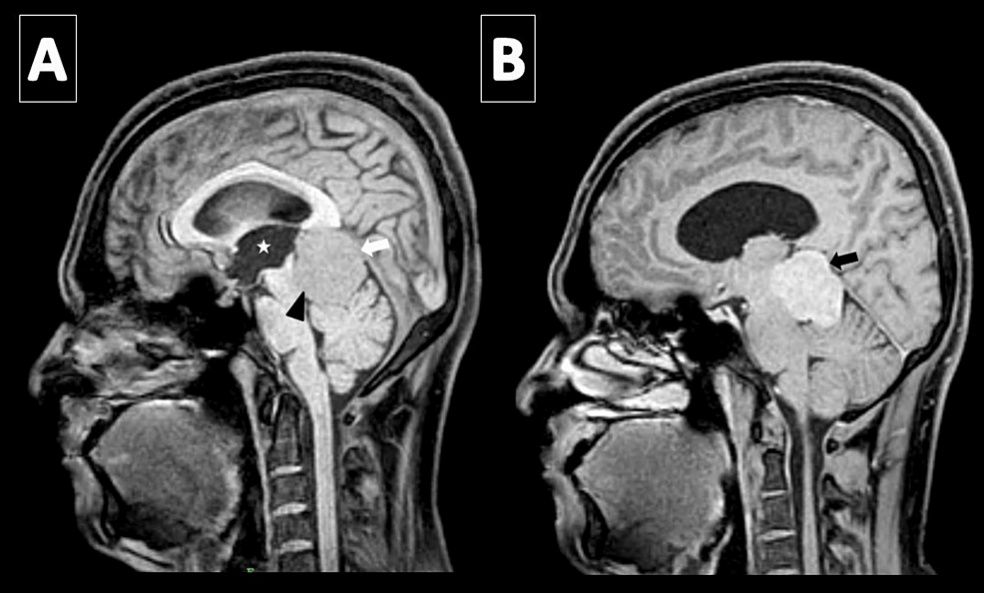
Case 1, a 40-year-old woman. Precontrast and postcontrast T1-weighted fat-suppressed sequences. (A) The sagittal precontrast T1-weighted image shows the infratentorial pineal region mass (white arrow) is isointense to gray matter and causes a mass effect on the tectal plate (black arrowhead) and cerebral aqueduct, resulting in mild obstructive upstream dilatation of the third ventricle (white star). (B) The sagittal postcontrast T1-weighted image shows the lesion homogeneously enhances (arrow).
